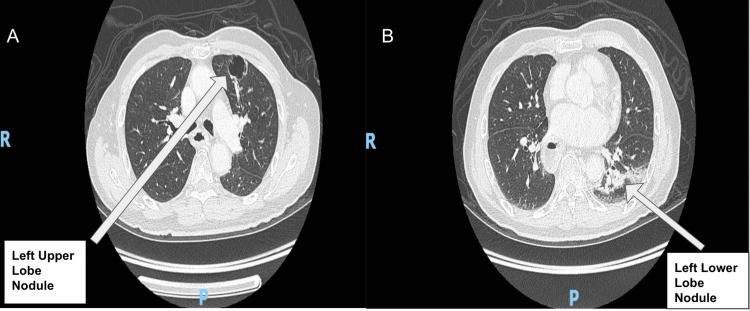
Computed tomography (CT) scan showing a 2.2 cm nodule in the left upper lobe and a 1.4 cm nodule in the left lower lobe.
